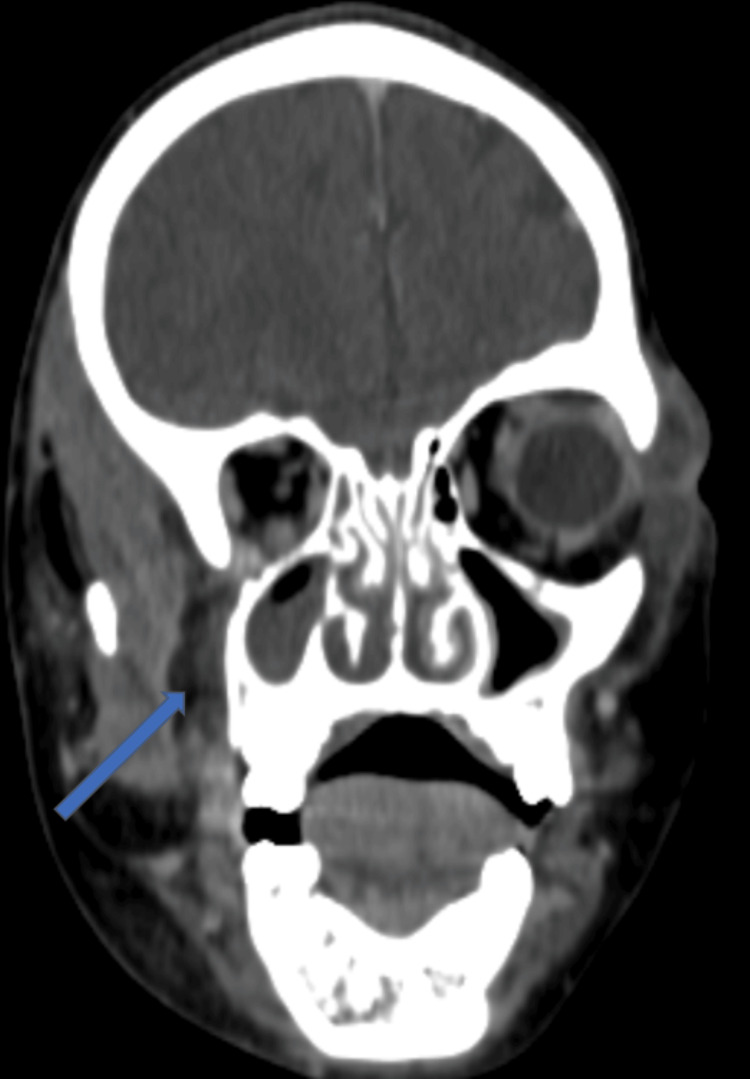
Coronal CT image showing right maxillary sinus opacification associated with infiltration of the intra- and extra-conal orbital fat (arrow) CT: computed tomography
